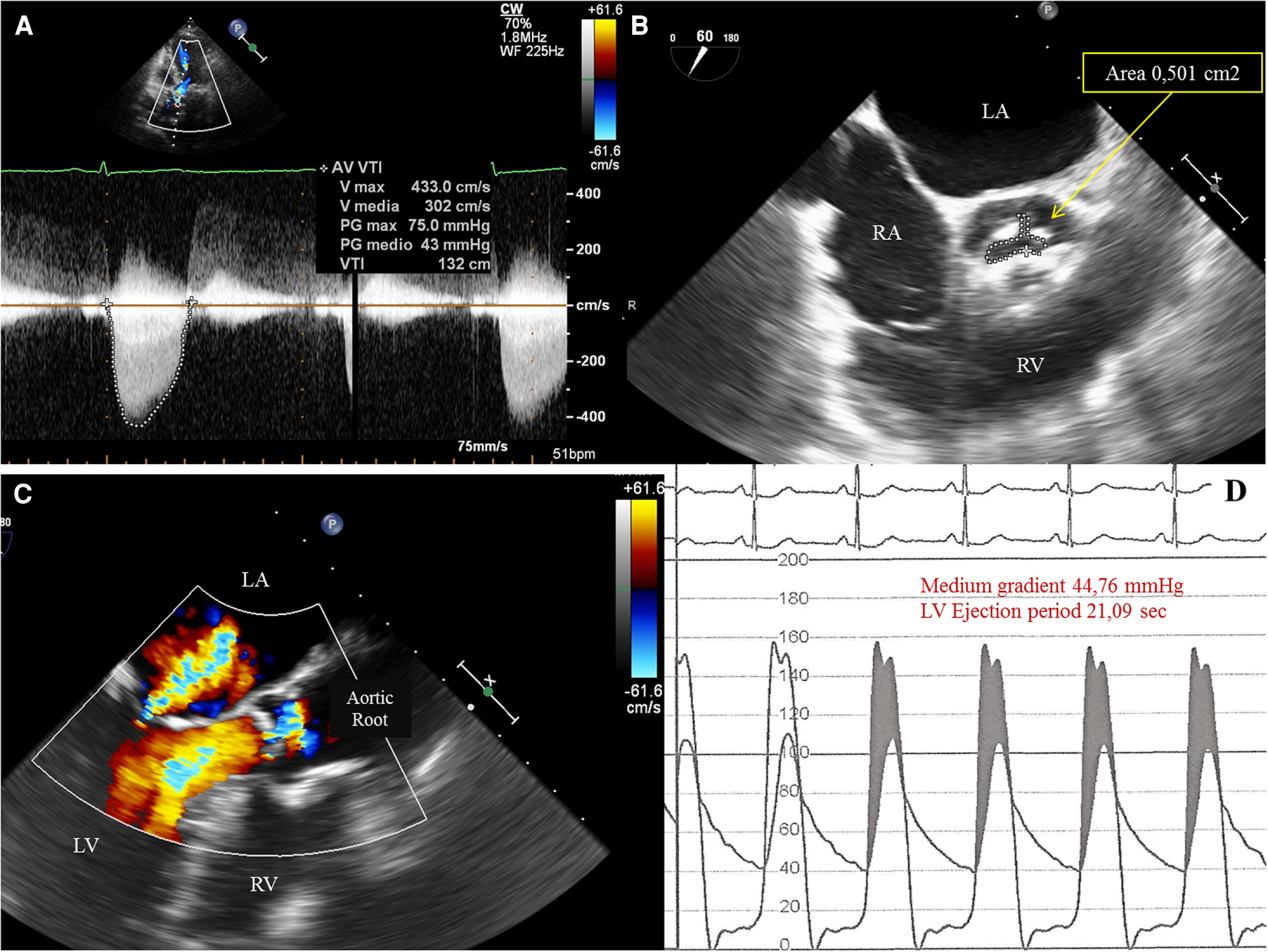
(A) Transthoracic echocardiography (TTE) 5-chamber view showing increased peak and medium transvalvular aortic pressure gradient of, respectively, 75 mmHg and 43 mmHg. (B)Transesophageal echocardiography (TEE) showing a tricuspid aortic valve with severe stenosis and a planimetric aortic valve area of 0,5 cm^2^. LA, left atrium; RA, right atrium; RV, right ventricle. (C) TEE showing a mild mitral valvular insufficiency with neither significant subvalvular and/or intraventricular pressure gradient nor evidence of systolic anterior motion (SAM) of the anterior leaflet of the mitral valve. (D) Invasive simultaneous registration of left ventricular (LV) and aortic pressures showing a significant transvalvular pressure gradient during the ventricular ejection period. LV pressure exceeds the aortic pressure (gray area, pressure gradient generated by valvular stenosis). The LV peak systolic pressure during ejection ranged between 152 to 156 mmHg and the aortic pressure between 102 to 106 mmHg. No elevated LV telediastolic pressure was documented.).
